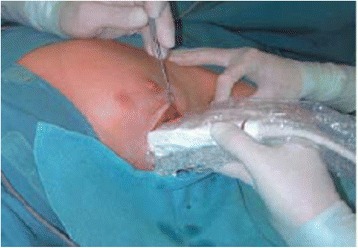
Intraoperative tumor localization by ultrasound
